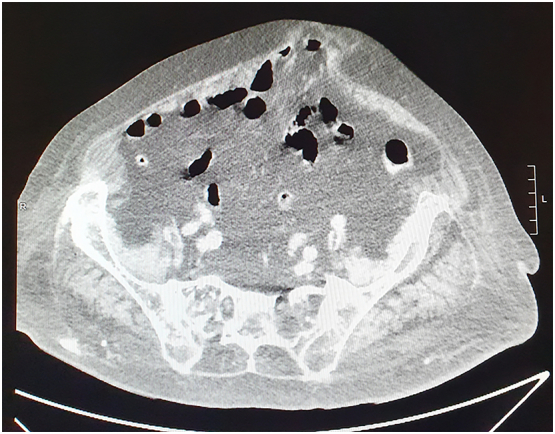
Preoperative abdominal computed tomography scan showing the protrusion of greater omentum and small bowel loop through the umbilical ring.
